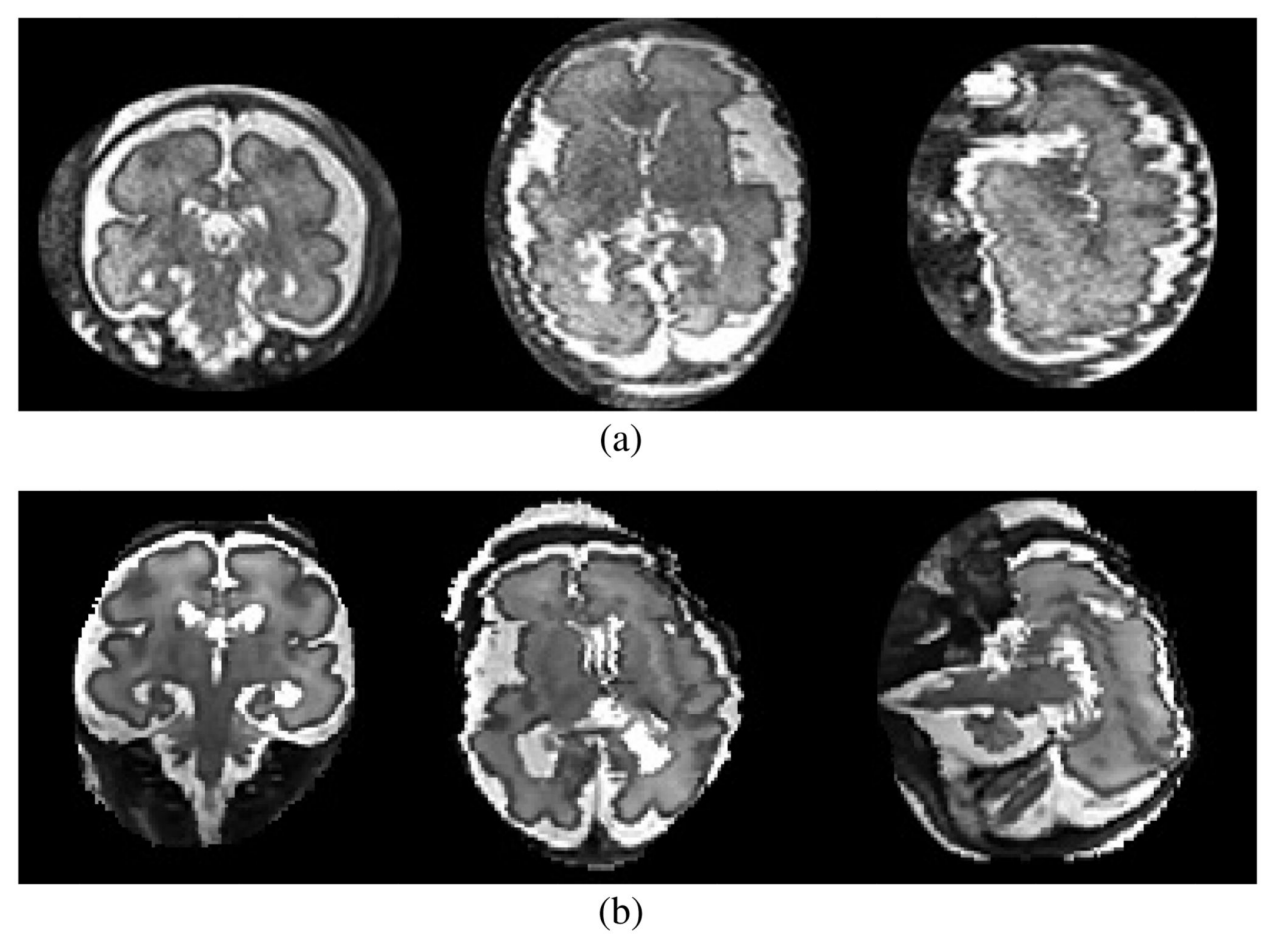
Results of the application of our method to three stacks of freehand 2D compound ultrasound (US). This dataset is reconstructed to 0.6 mm isotropic voxel size and contains 568×406×630 voxels. The investigated area in red shows the vessel tree of a volunteer’s liver. (a-c) show a multi-planar reconstruction of the compounded average [3] of the input slices resampled in a joint volume with 0.6 mm isotropic voxel size. (d) gives an overview over two of the acquired 2D sweeps in 3D. (e) shows the original data, (f-k) show the resulting reconstruction in three orthogonal orientations comparing the average of the image data to the result of our super-resolution (SR) framework.
